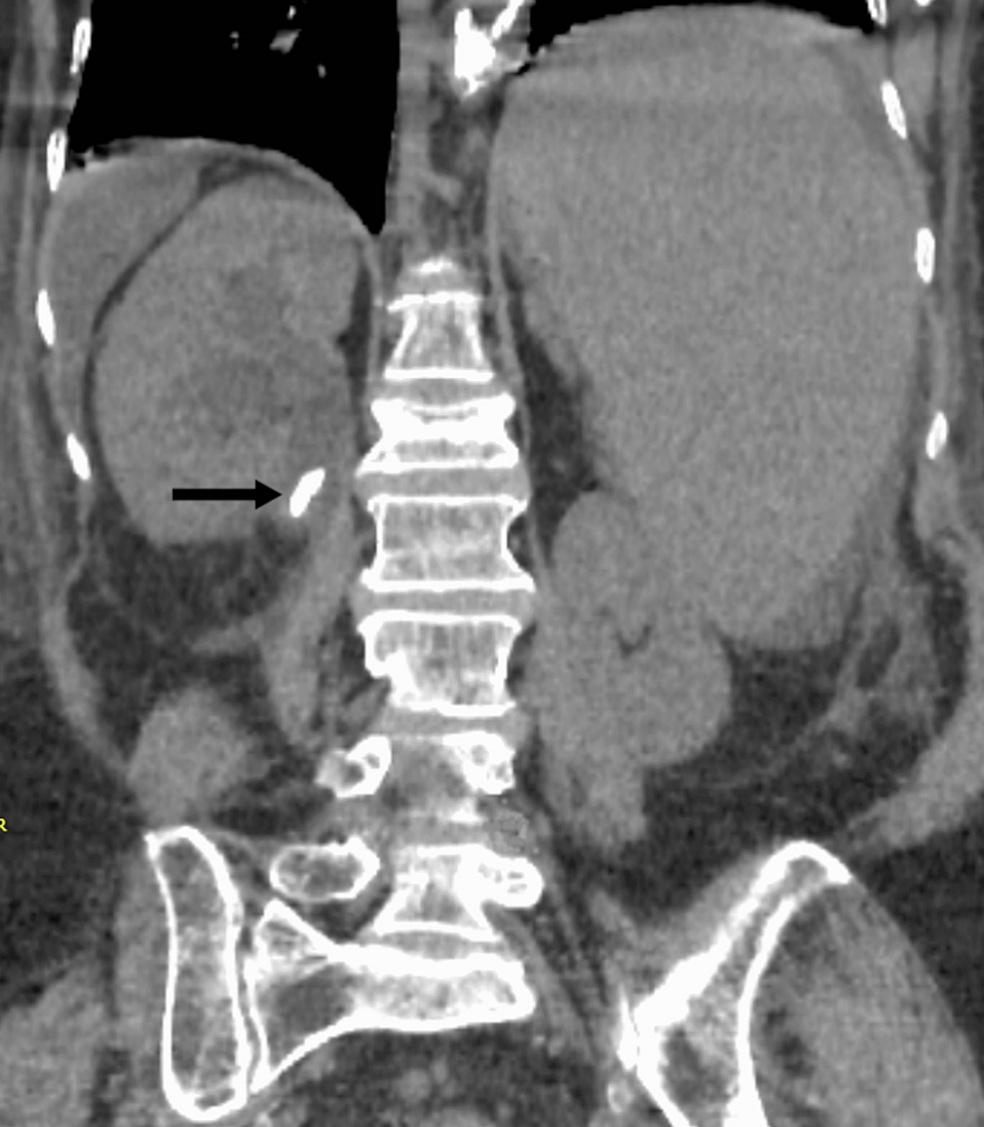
Non-contrast CT scan showing calcific calculus in right pelvic-ureteric junction measuring 15mm x 5mm with right hydronephrosis CT: computed tomography
